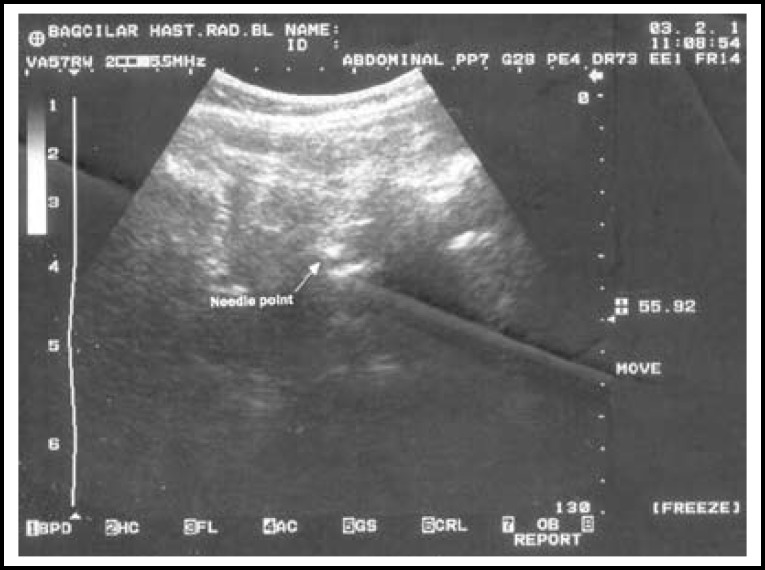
Ultrasonic image of the needle point
